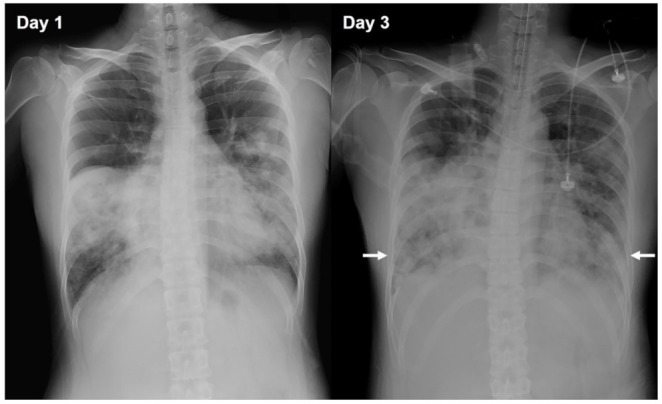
Chest radiographs showing the progression of pneumonia. Chest radiograph on day 1 (left) and day 3 (right). The radiograph performed on day 3 shows the progression of the pneumonia, with patchy opacification in the lower lobes of the lungs (arrows).
